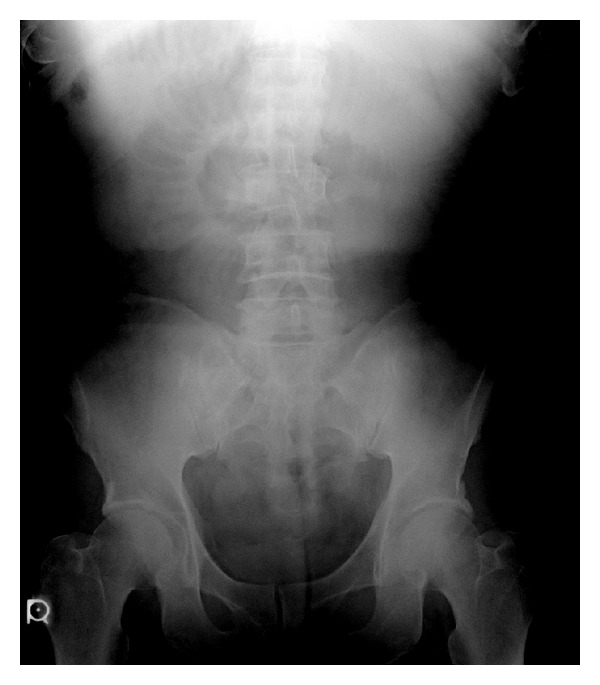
A plain abdominal X-ray (in decubitus) revealed a prominent sentinel loop.
